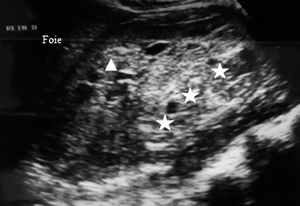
Ultrasound image of a large hematoma under the capsule of the right kidney. Heterogeneous hyperechoic formation (white stars) in median subcapsular position pushing off the right kidney (white triangle).
